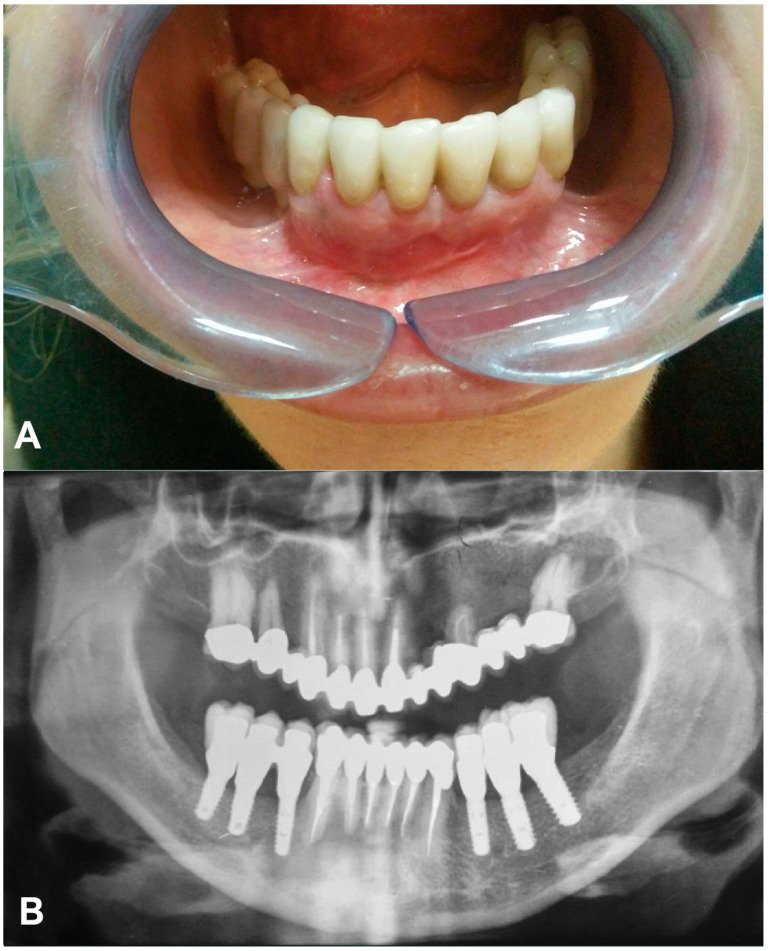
Five month follow-up. (A) Intraoral view; (B) Orthopantomography.
